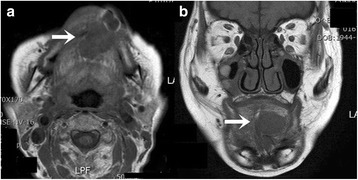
T1-weighted magnetic resonance image showing a well-defined heterogeneous lesion (*white arrow*). a Axial view. b Coronal view
